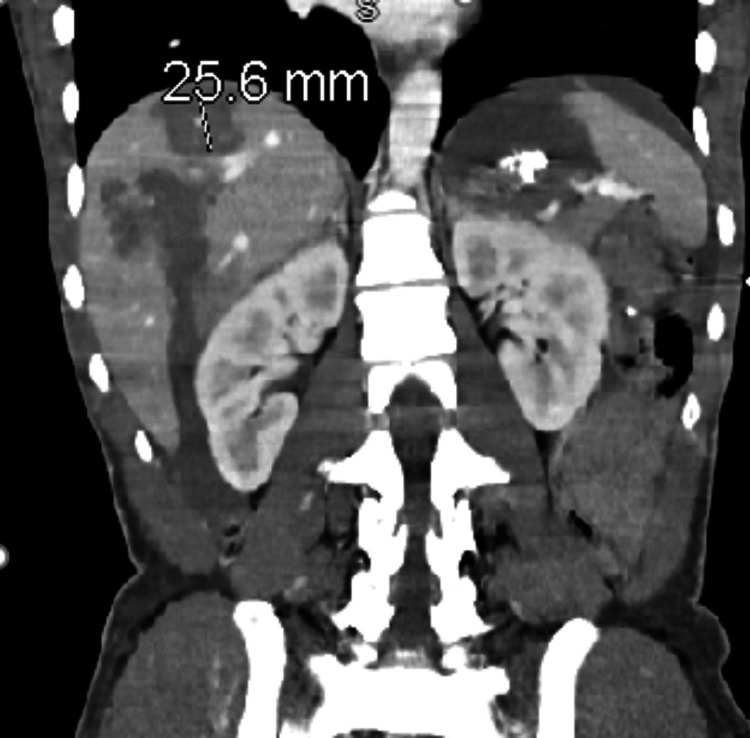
CT abdomen multiple lacerations in the right lobe of the liver largest measuring approximately 5.7 cm in depth
